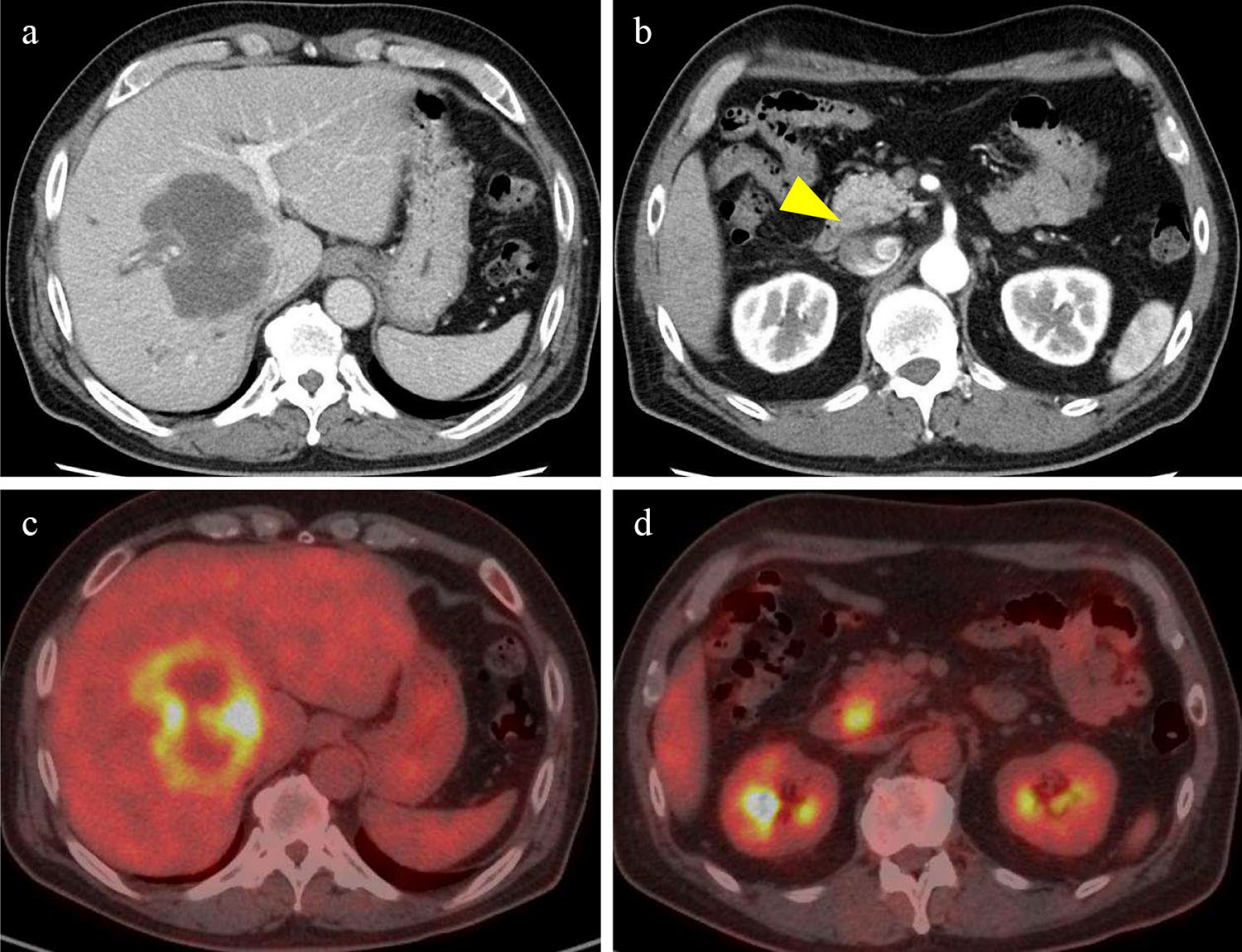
Contrast-enhanced computed tomography (CT) (a, b) and positron emission tomography-CT (c, d) images. a Intrahepatic cholangiocarcinoma with minimal enhancement in the right lobe of the liver. b Swollen lymph nodes are observed behind the pancreatic head. c Fluorodeoxyglucose (FDG) collection is observed in the tumor of the right lobe of the liver. d FDG accumulation is observed in the lymph nodes of the pancreatic head, which is considered a metastasis
